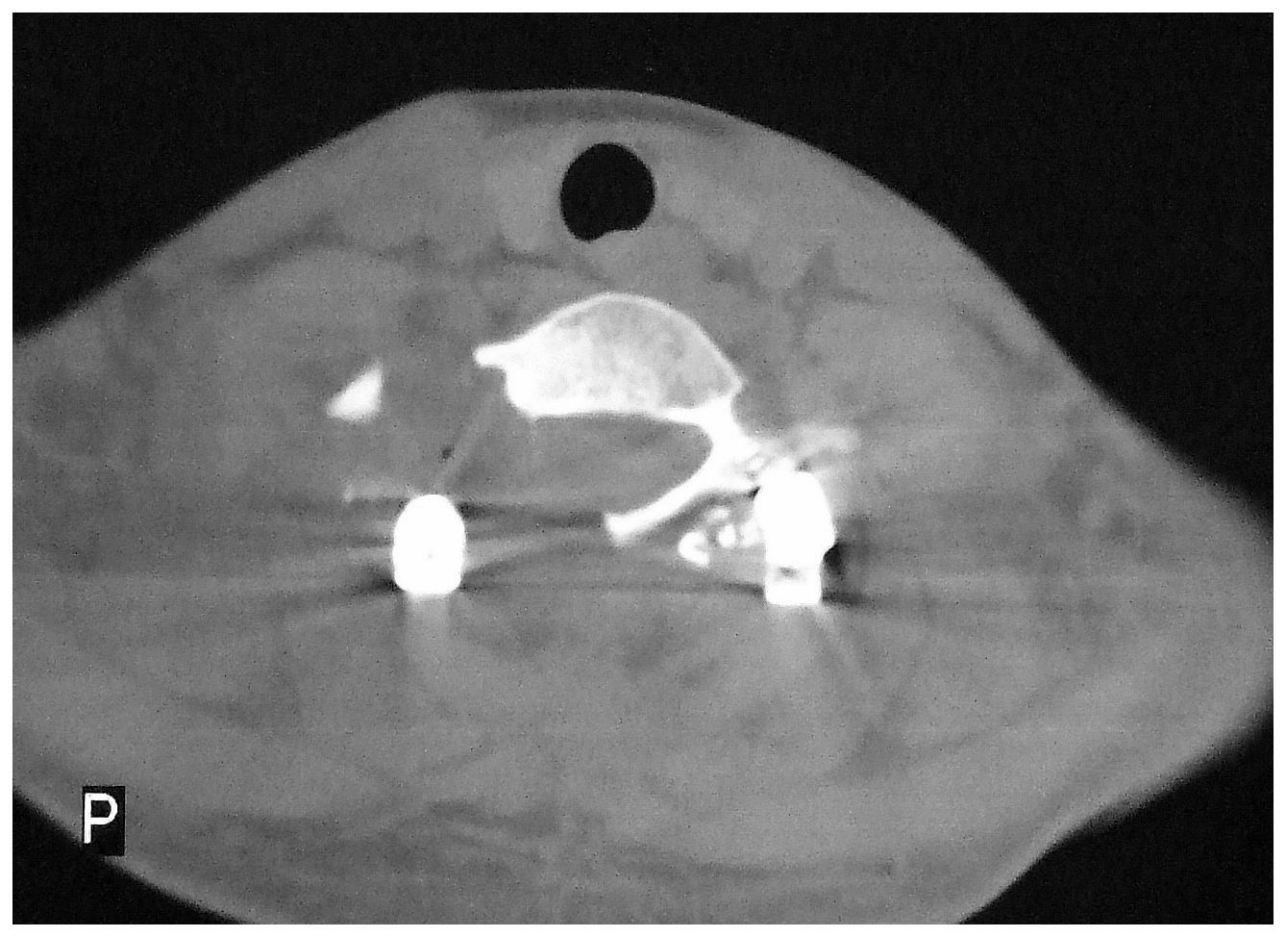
Postoperative CT scan showing the extents of en bloc resection of the tumor and decompression.
